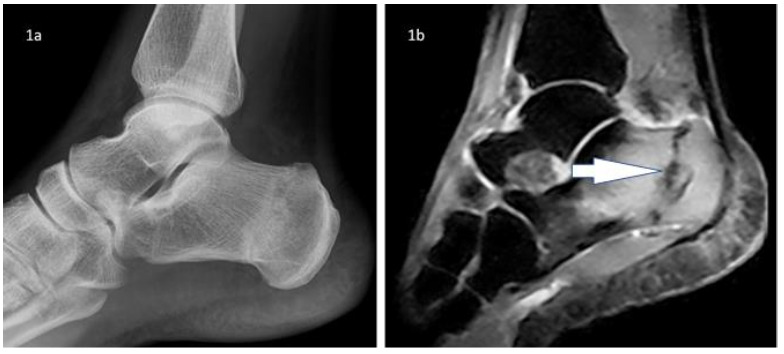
Lateral X-ray showing oblique stress fracture of the calcaneus with surrounding sclerosis.
